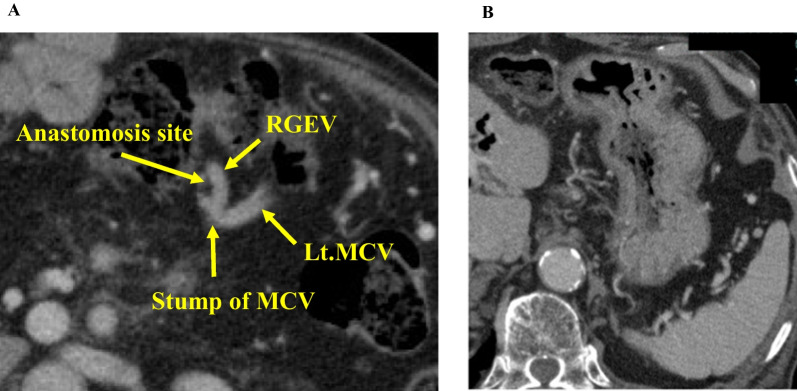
CT after the surgery. A CT 2 years after the surgery showing the patency of the RGEV–MCV anastomosis. B CT 2 years after the surgery showing the absence of varices around the stomach. *CT* Computed tomography, *RGEV* Right gastroepiploic vein, *MCV* Middle colic vein
